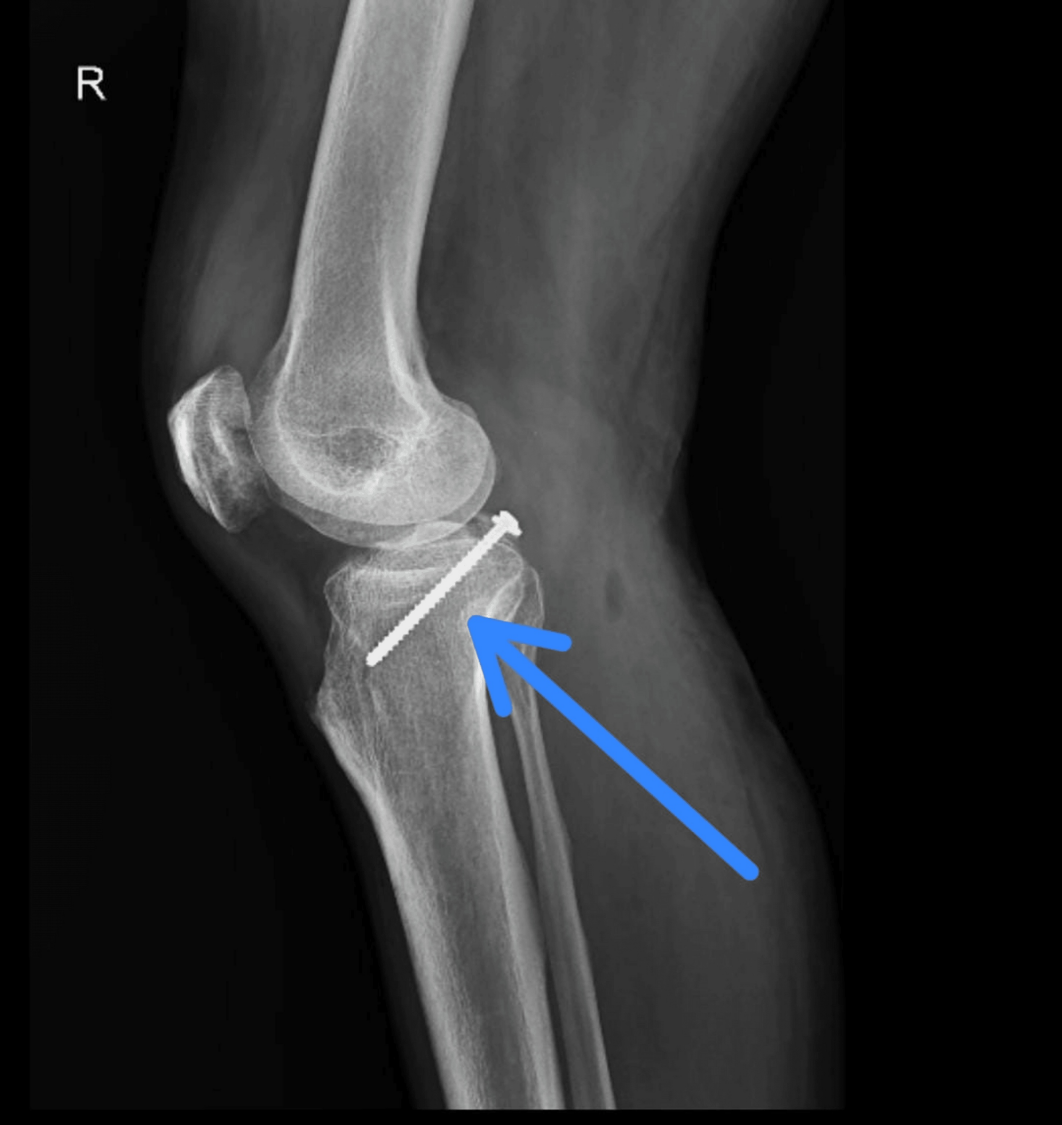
A lateral view of the X-ray shows a right knee with a screw used to fix an avulsion of the posterior cruciate ligament (PCL).
